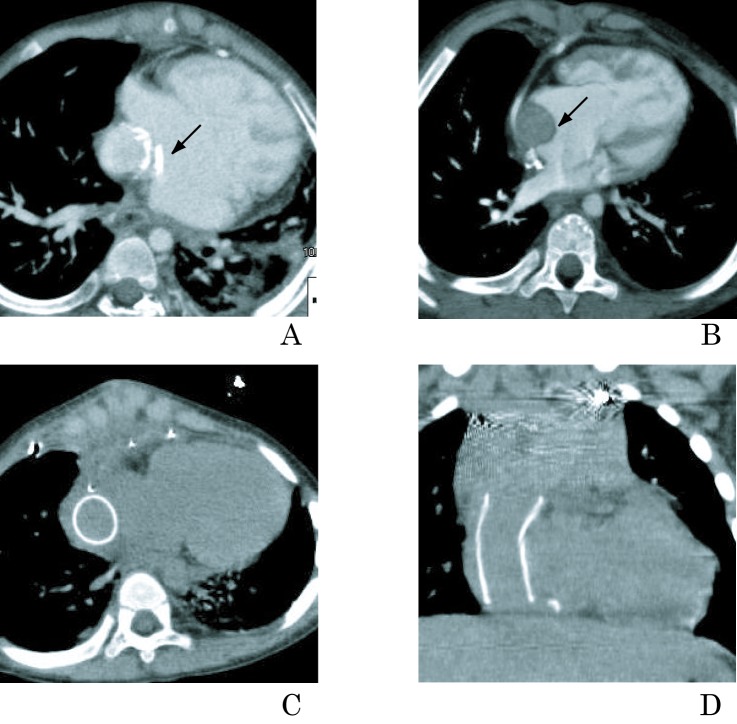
Multidetector-row computed tomographic views of polytetrafluoroethylene (PTFE) grafts used for atrial septal patches of Fontan procedure and extracardiac conduit of total cavopulmonary connection (TCPC). (A) Four-year-old girl with single ventricle who underwent lateral tunnel Fontan operation. Calcified intimal hyperplasia (arrow) is revealed on both sides of the atrial PTFE patch. (B) Four-year-old boy with DORV who had undergone lateral tunnel Fontan modification. Atrial septal patch (arrow) is clearly shown; no calcification is detected. Four-year-old boy with single ventricle who underwent extracardiac TCPC. Unenhanced MDCT was performed. The PTFE graft conduit is clearly shown with homogeneous high density in the axial (C) and coronal (D) views. *Reproduced with permission from American Heart Journal 2007; 153: 806.e1-8, Mosby, Inc.*
